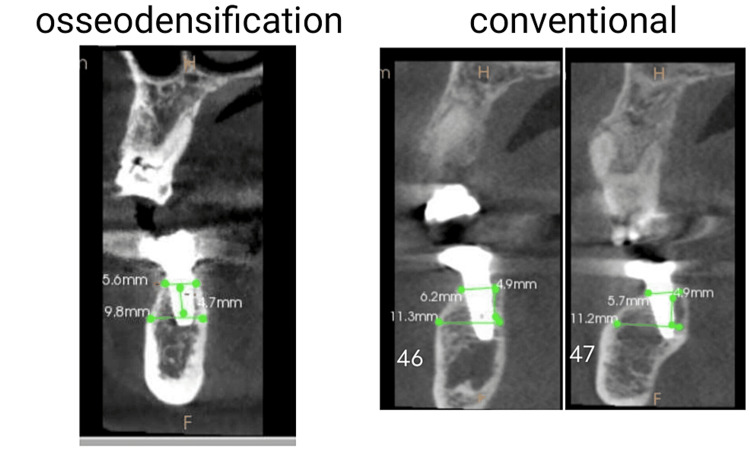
Six-month postoperative cone-beam computed tomography to evaluate alveolar ridge expansion.
